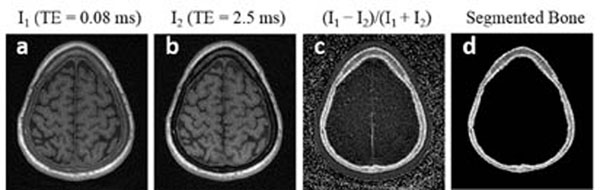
MR images reconstructed from a dual-echo 3D scan of a human head, showing the potential for skull imaging and segmentation using UTE MRI. Imaging resolution is 1.25 mm isotropic.
